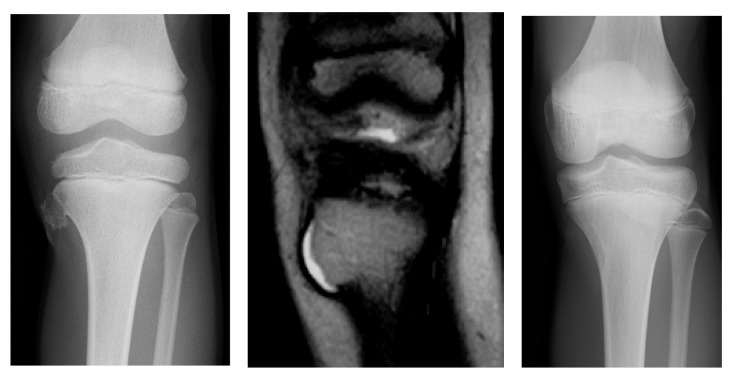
A radiograph revealing a pedunculated osteochondroma at the proximal tibia (left). The thickness of the cartilage cap is 2.83 mm upon MRI examination (middle). After 6 years, the tumor vanished, as depicted by a radiograph (right).
